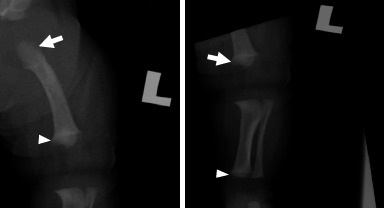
A: Left femoral radiograph showing proximal femoral lucency (arrow) and distal femoral metaphyseal cupping (arrowhead). B: Left tibial/fibular radiograph showing distal femoral metaphyseal cupping (white arrow), long fibula bone and distal tibial metaphyseal cupping (arrowhead).
